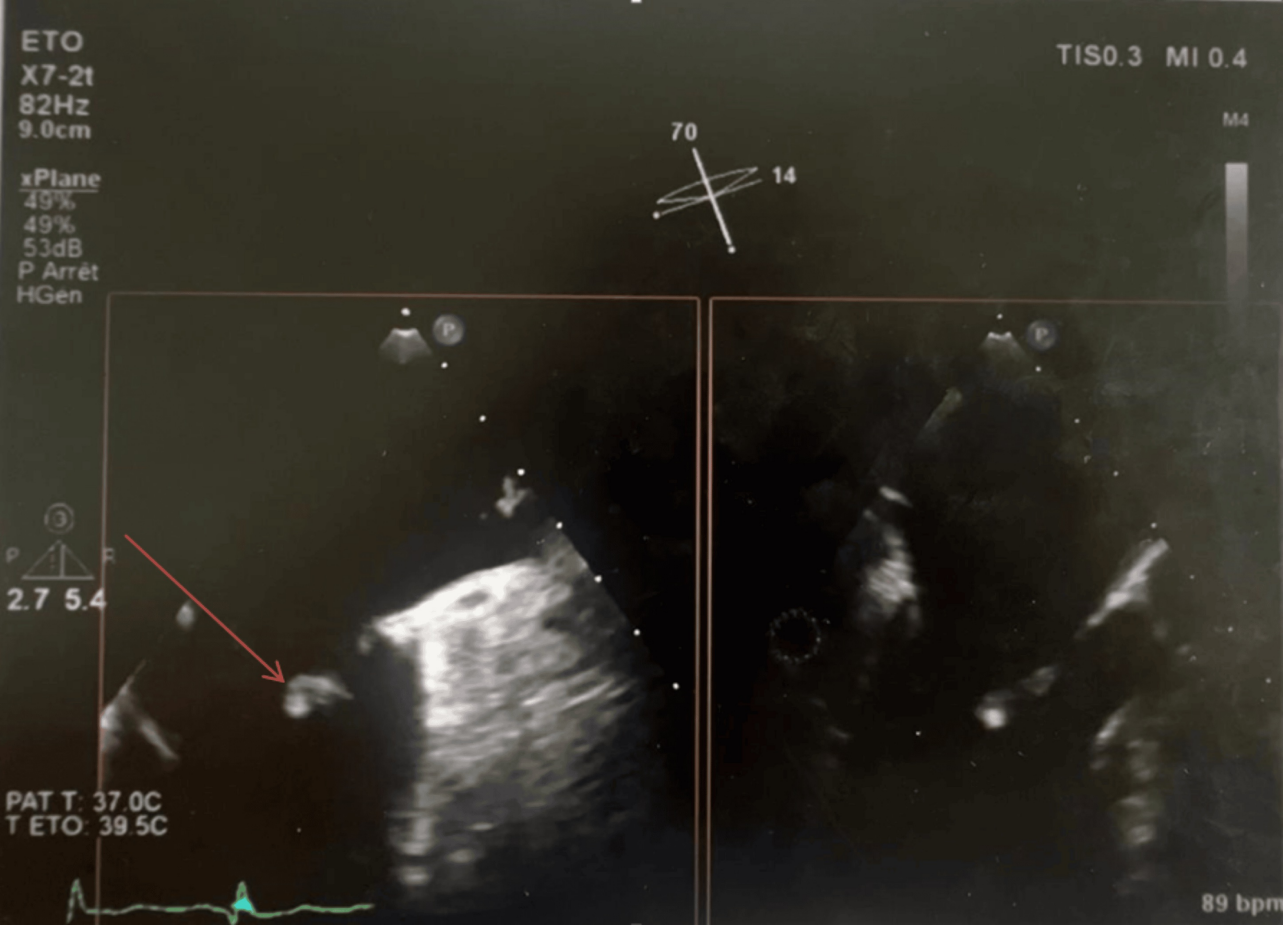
Two-dimensional transesophageal echocardiography showing, in two views, a mobile pedunculated mass attached to the anterior mitral leaflet (arrow).
